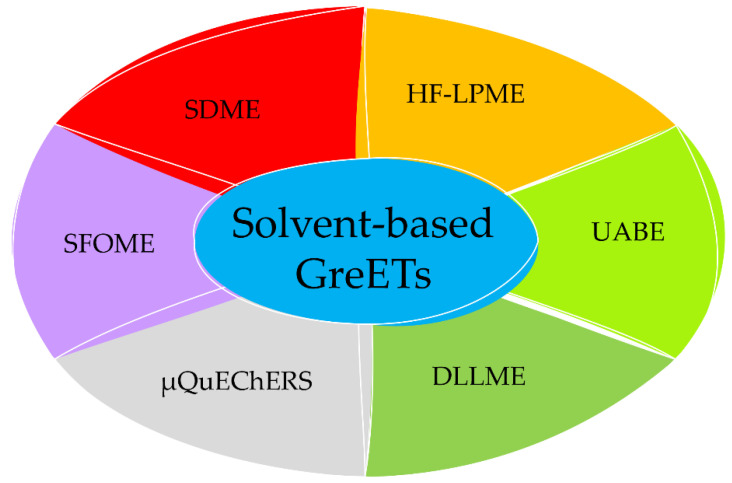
Different liquid-based GreETs used in several fields of analysis. Legend: DLLME: dispersive liquid–liquid microextraction; GreETs: green extraction techniques; HF-LPME: hollow fiber liquid-phase microextraction, SDME: single-drop microextraction; SFOME: solidification of floating organic drop microextraction; UABE: ultrasound-assisted back extraction; µQuEChERS: micro-QuEChERS.
